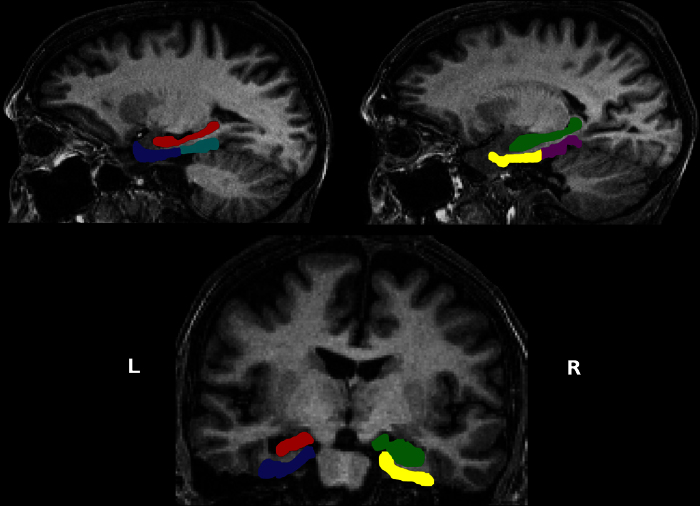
Brain regions of interest. The top panel shows sagittal views through the left and right hemispheres, and the lower panel a coronal section, from the structural MRI scan of one of the patients with left HS; L = left side of the brain, R = right side of the brain. The left hippocampus (sclerosed) is coloured in red, the right hippocampus in green, the left EPC in blue, the right EPC in yellow, the left PHC in turquoise, and the right PHC in magenta. (For interpretation of the references to colour in this figure legend, the reader is referred to the web version of the article.)
